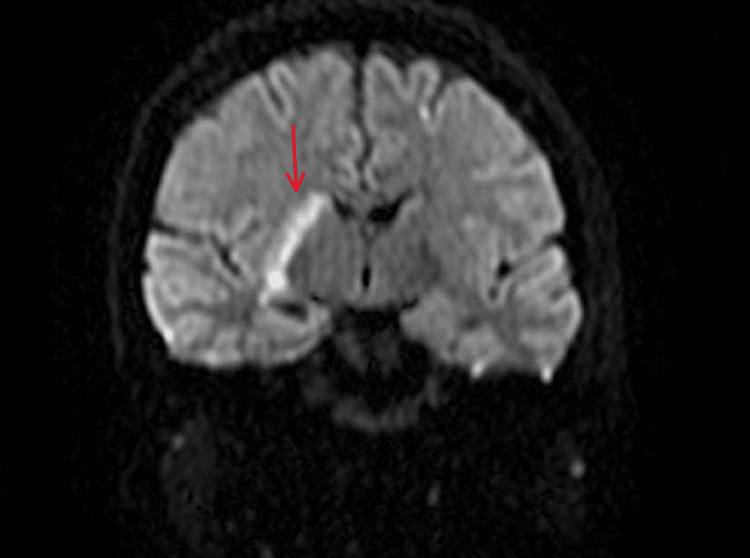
MRI of the brain showing diffusion restriction in the basal ganglia on the right side. MRI = magnetic resonance imaging
